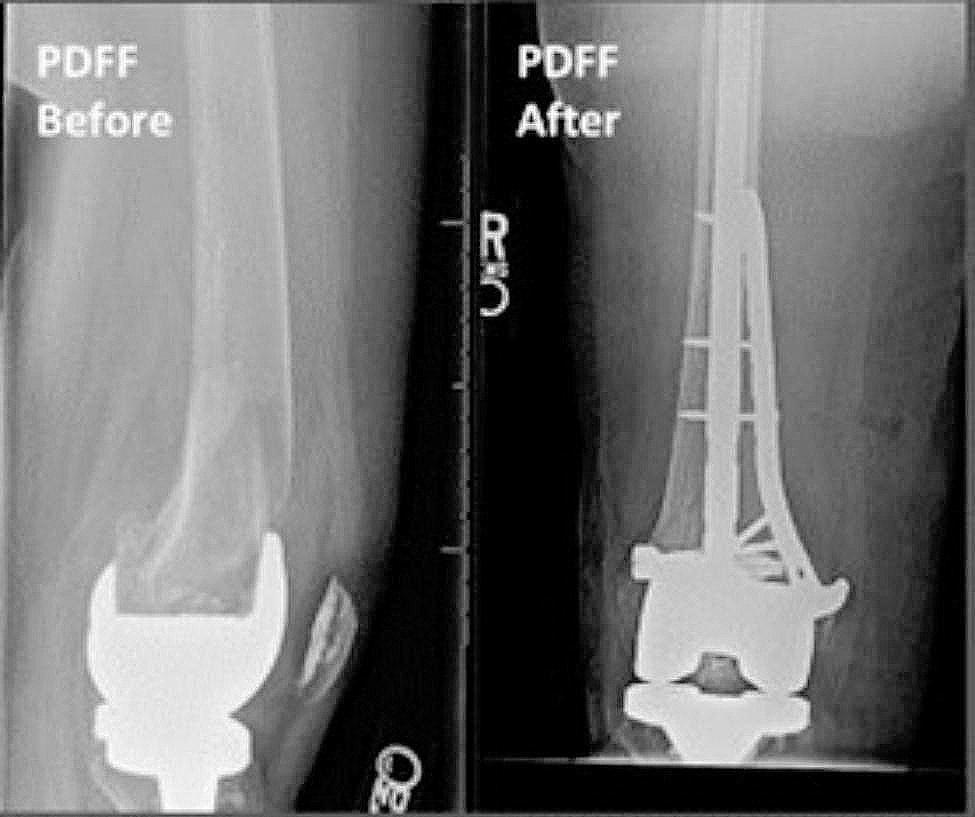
Preoperative (left) and post-NPC (right) PDFF radiographs
